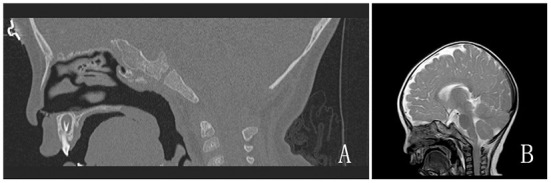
(A,B) Preoperative imaging examinations. (A) Temporal bone CT; (B) Brain magnetic resonance imaging.
